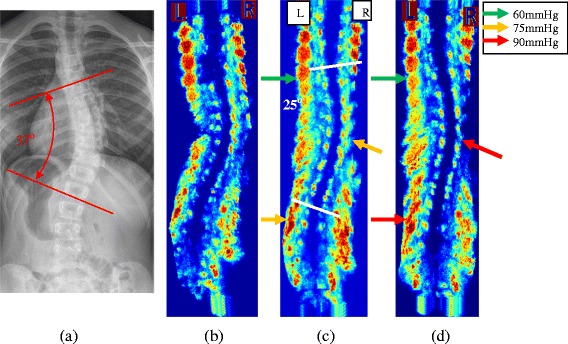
X-ray and US Images of a brace patient. a) The standing pre-brace x-ray with Cobb angle 37°, b) the baseline US scan, c) the 1st trial US scan with Cobb angle 25° and d) the 2nd trial US scan with Cobb angle 23. The color arrows indicate the pressure magnitude applied to the body.
